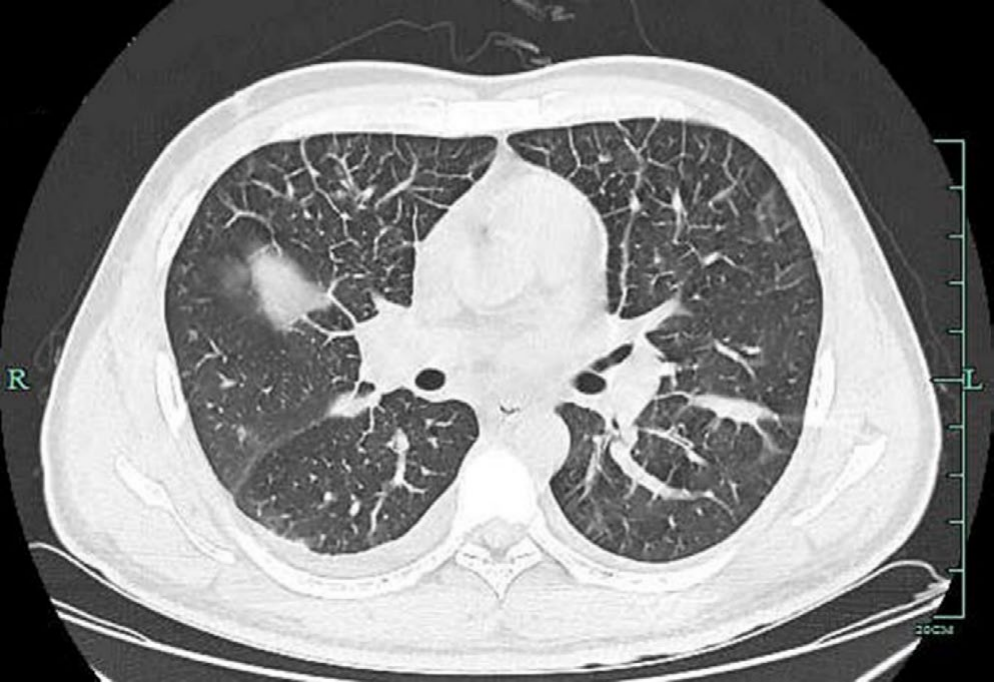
HRCT: Diffuse interstitial pulmonary edema and alveolar effusion in both lungs, with a small amount of fluid in the right pleural cavity.
